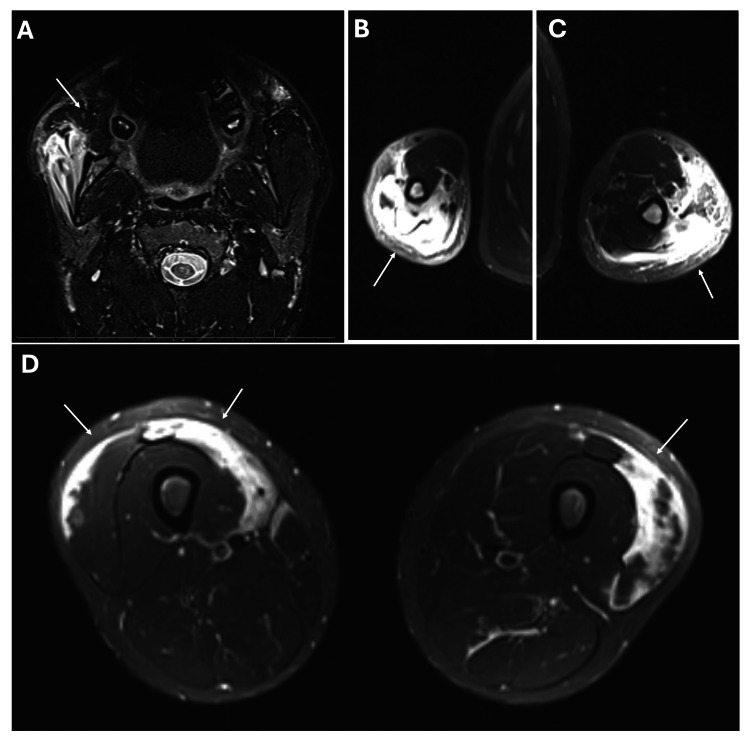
MRI of the facial, humeral and femoral muscles. (A) MRI of the facial muscles revealed thickening, edema, and enhancement of the right masseter muscle (arrow). (B and C) MRI of the upper extremities showed extensive intramuscular, fascial and subcutaneous edema in the triceps (arrows). (D) MRI of the thighs revealed diffuse muscle edema was evident in bilateral vastus lateralis and rectus femoris (arrows).
